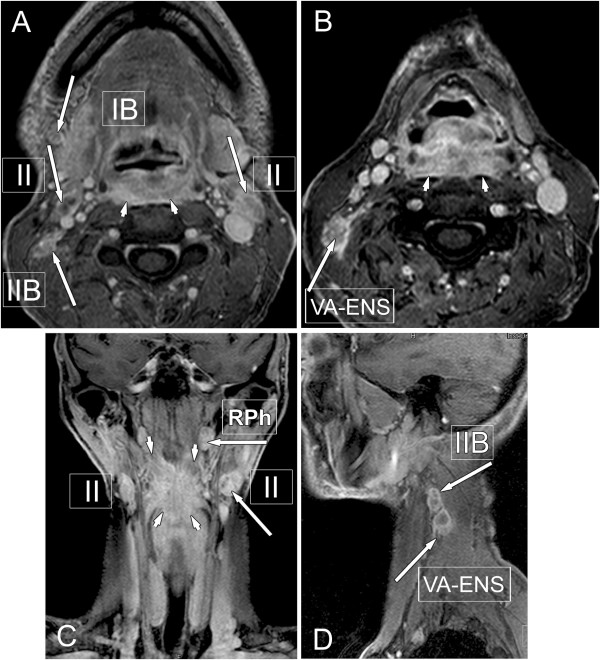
Metastatic lymph nodes. A, B, C, D figures: post-contrast T1-weighted MRI images with fat suppression showing carcinoma of the dorsal pharyngeal wall (arrow-heads) with signs of retropharyngeal (RPh) tumor spread manifesting in bilateral retropharyngeal lymphnode enlargement above the upper level of tumour (large arrow on “C” image). Long arrows show metastatic lymph nodes in different cervical regions, with necrotic center, and irregular margins as typical sign of extranodal tumour spread (ENS).
